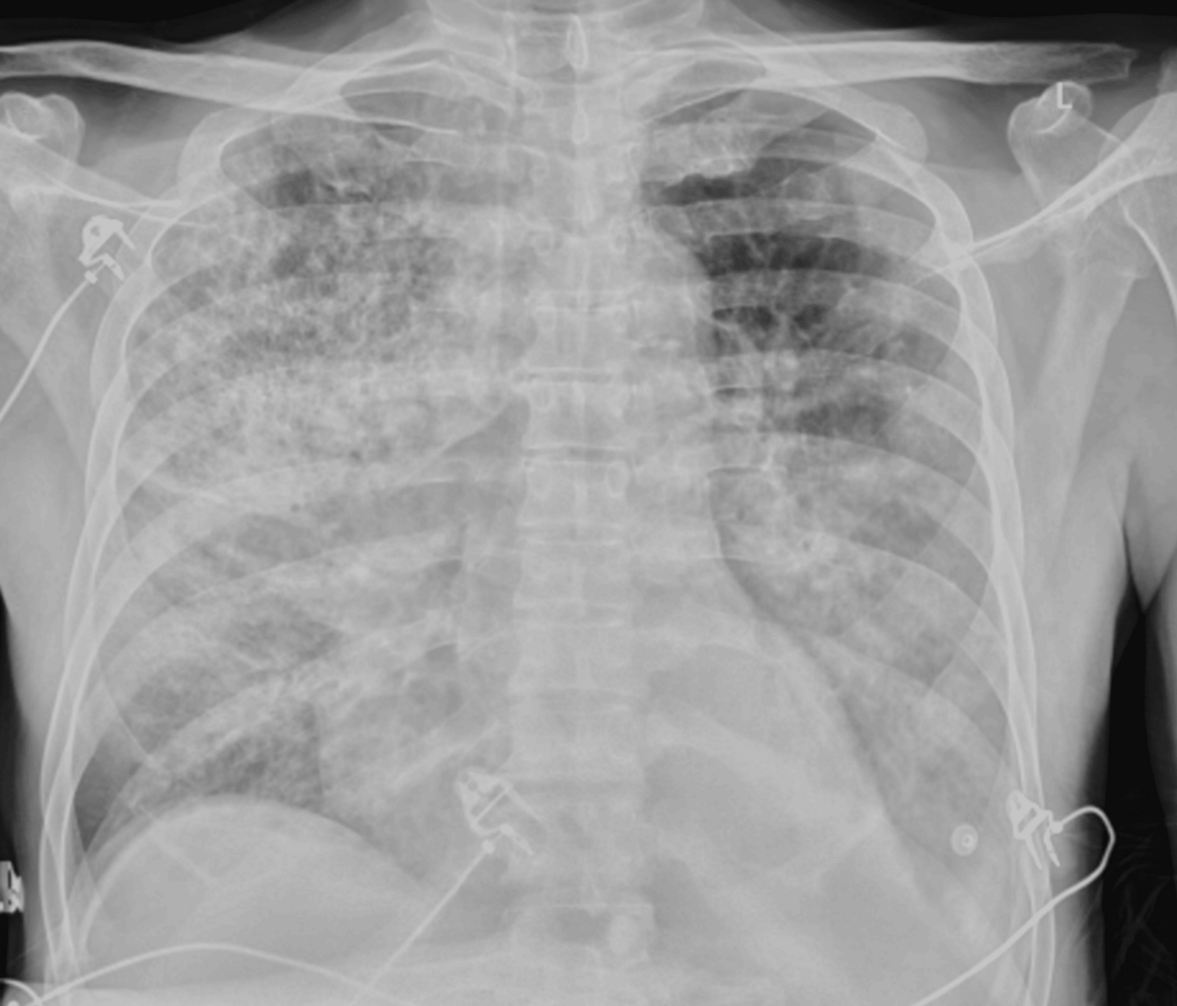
Chest X-ray on the sixth day of hospitalization indicates bilateral progression of fine mottled changes, which was accompanied by clinical deterioration and the need for reintubation.
